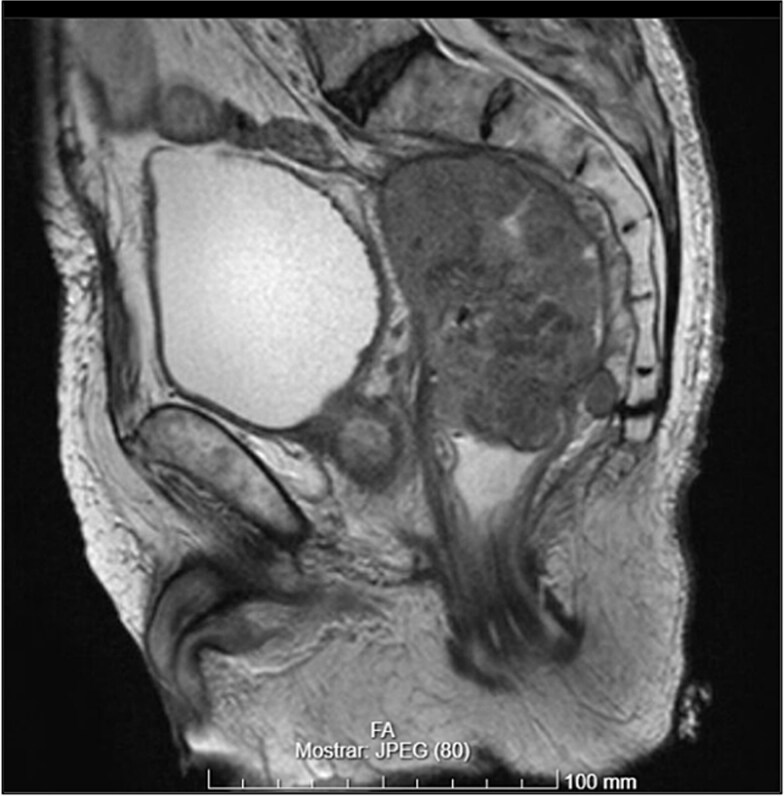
Pelvic recurrence (MRI—T2 sagittal plane).
